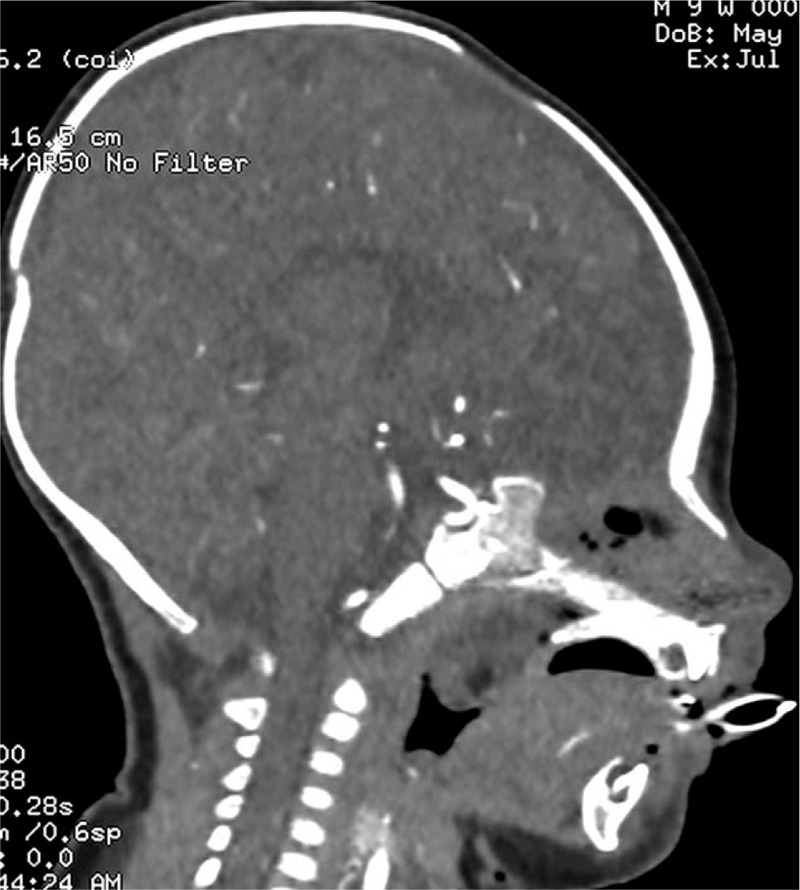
Nasopharyngeal contrast-enhanced computed tomography showing that the large wing of the left sphenoid bone is thinner and less continuous than normal; the inner plate of the left wing is not shown.
